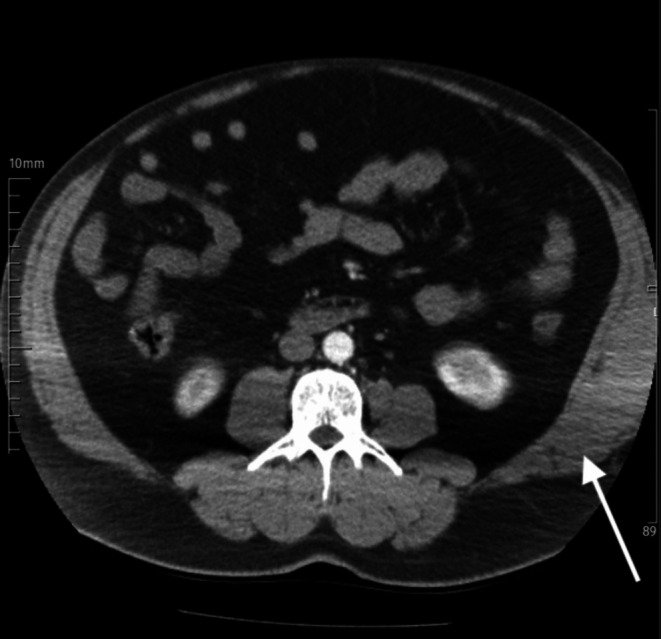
Follow‐up computed tomography performed 2 weeks later showing that the size of the hematoma decreased (arrow).
